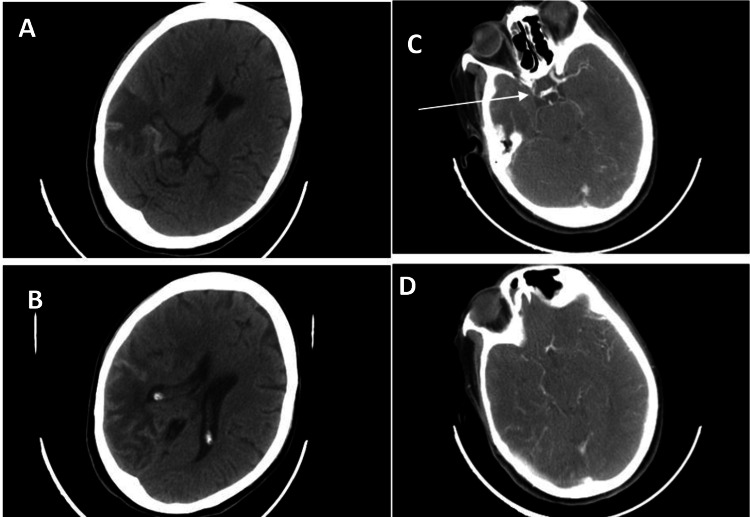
(A, B) CT scan: right temporo-parietal hypodense area with peripheral cortical hyperdensity, suggestive of a right middle cerebral ischemic stroke with resolving hemorrhagic transformation. (C, D) CT angiography: reduction in the caliber of the right carotid artery, stopping at the level of the intracranial internal carotid artery, with the absence of visualization of the proximal segments of the right anterior and middle cerebral arteries CT: computed tomography
